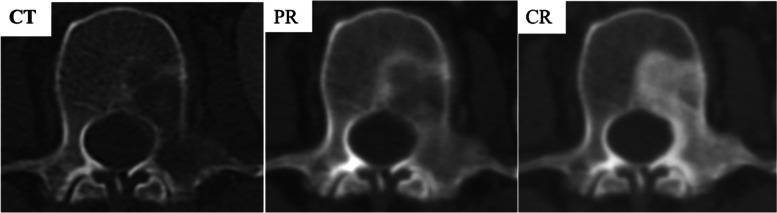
Representative computed tomography (CT) scans demonstrate evaluation criteria. CT scans obtained before treatment shows lytic metastasis. After some treatment, the scans showed “partial filling in,” categorized as partial response (PR), and “complete filling in,” categorized as complete response (CR)
